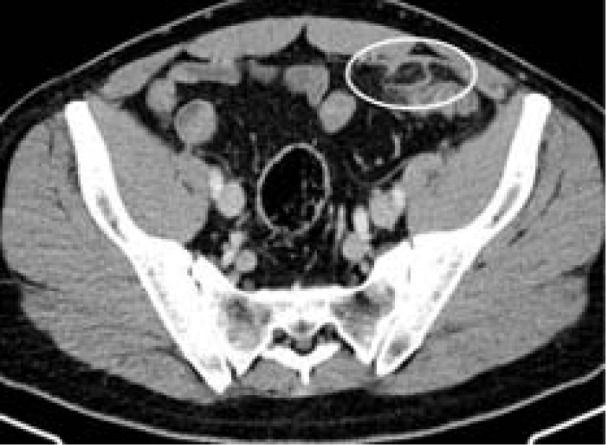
Contrast enhanced axial abdominal CT demonstrating primary epiploic appendagitis adjacent to the sigmoid colon 7 months prior to presentation
